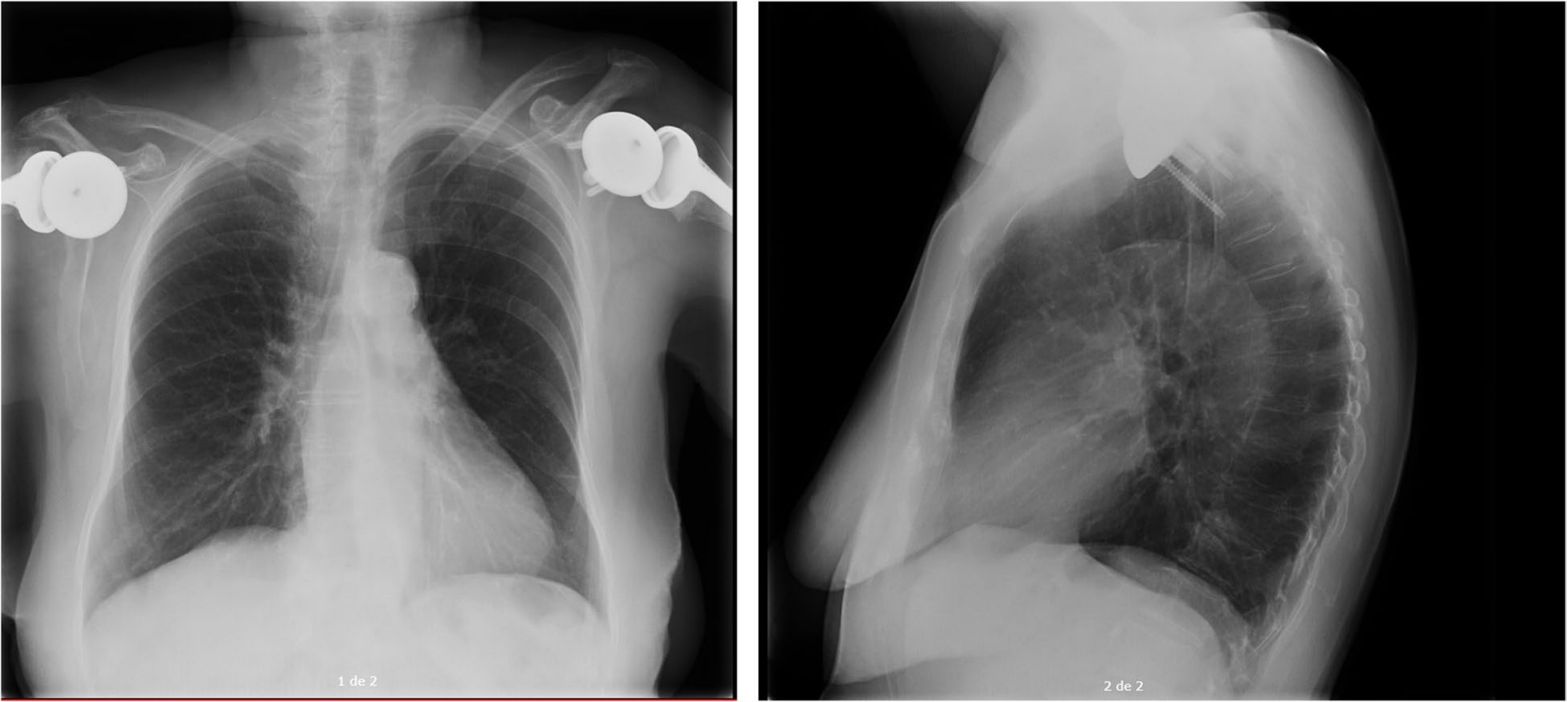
Posteroanterior and lateral chest X-ray after treatment with resolution of pleural and pericardial effusion
